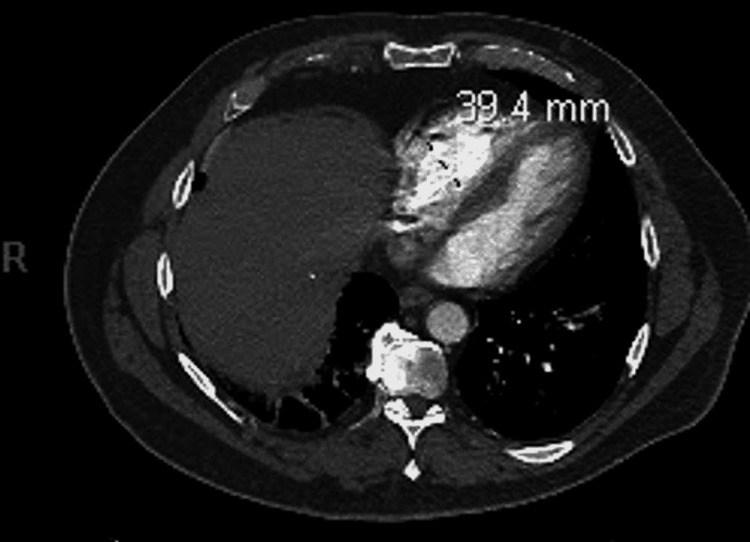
CT pulmonary angiography showing bilateral pulmonary emboli, with a larger thrombus on the right.
